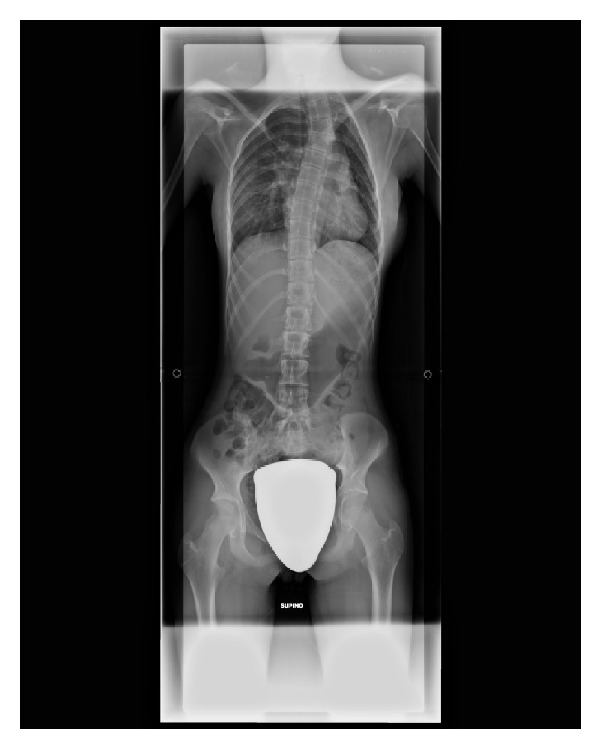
Chest radiograph (CXR) image. CXR shows a left cervical and right dorsal scoliosis with left deviation of sternum.
